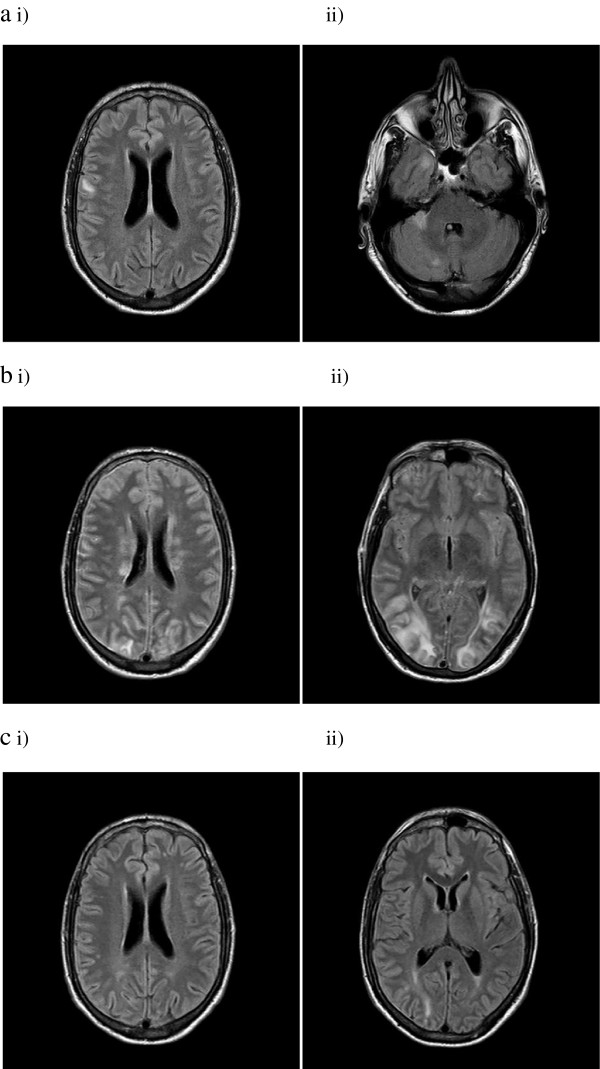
Sequential T2- weighted FLAIR MRI Brain imaging. a) Nodularly enhancing lesions on initial presentation in the i) right anterior temporal cortex and ii) right cerebellum. Subcortical U-fibre high signal intensity lesion in the right frontoparietal area as shown by T2- weighted FLAIR MRI. b) Marked interval increase in the signal hyperintensities demonstrated by T2- weighted FLAIR MRI in i) frontal, parietal and temporal lobes and the ii) cortical and subcortical/deep white matter of the occipital lobes following acute deterioration and consistent with PRES. c) Interval reduction of signal hyperintensities as shown by T2- weighted FLAIR MRI in i) parietal and ii) occipital lobes demonstrating resolving vasogenic oedema.
